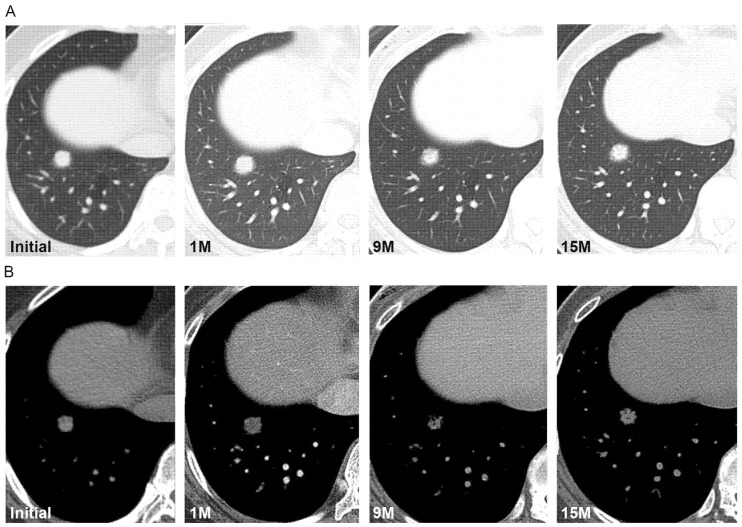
(A,B) Axial CT scans of the right lung in a 52-year-old female demonstrate a part-solid nodule that fluctuates in size and density during the 15-month follow-up. Initial CT scan demonstrates a part-solid nodule in the right lower lobe with a diameter of 14 mm. One month follow-up CT scan demonstrates the increased size of the nodule (16 mm). Nine-month follow-up CT scan demonstrates the decreased density and size (14 mm) of the nodule; fifteen-month follow-up CT scan demonstrates the increased density but no change in the nodule size. Surgical biopsy results confirmed the diagnosis of solitary pulmonary capillary hemangioma.
